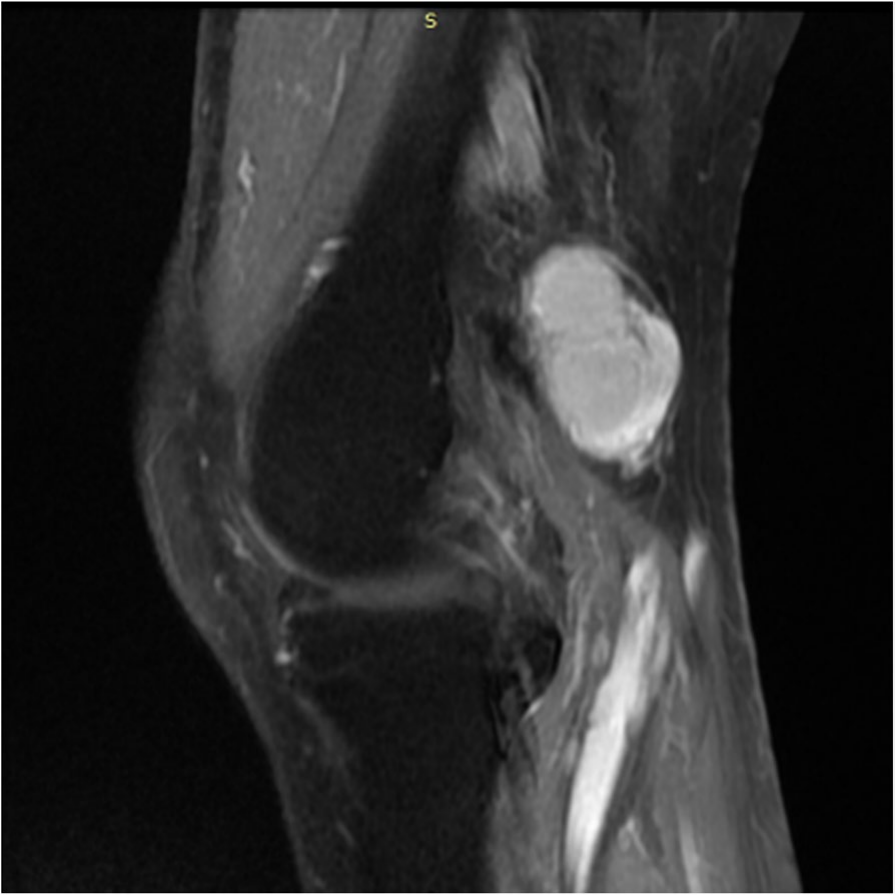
MRI fat-suppressed gadolinium-enhanced T1-weighted image (sagittal and Coronal view) show a sharply demarcated mass with avidly homogeneous enhancement
